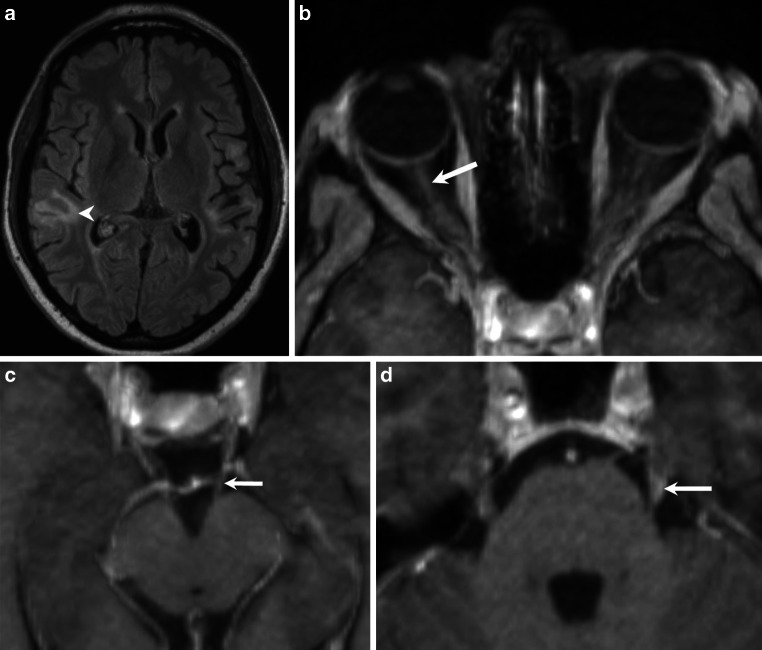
On axial FLAIR images (a) obtained 10 months later, the right-sided temporal lesion appears less pronounced (*arrowhead*). Axial T1-weighted postcontrast images (b–d) acquired during the same imaging session reveal contrast enhancement of the optic nerves (b, *arrow*), the oculomotor nerves (c, *arrow*), and the trigeminal nerves (d, *arrow*)
